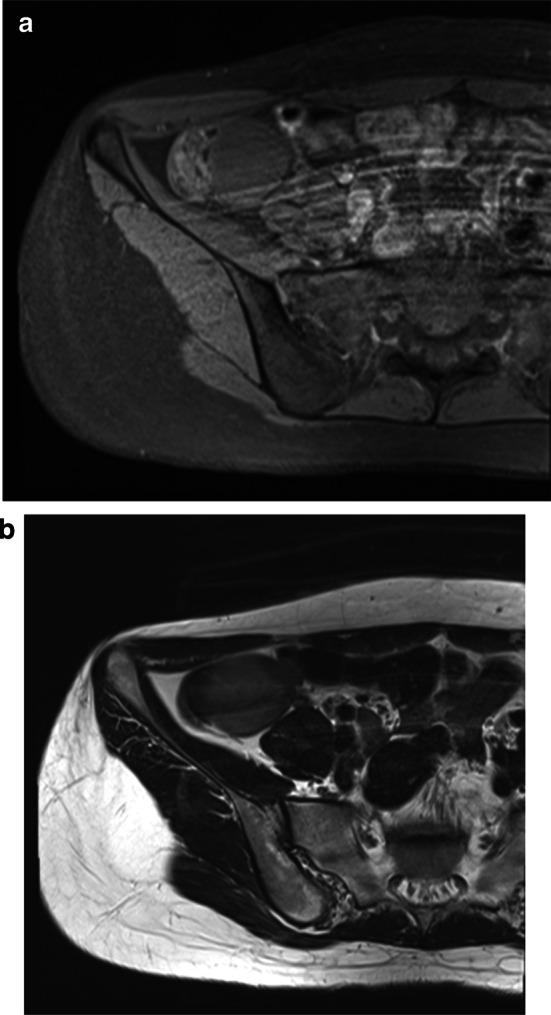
Abdominal MRI. MRI reveals a 56 × 43-mm mass attached to the cecum, appearing hypointense on T1-weighted imaging (a) and hyperintense on T2-weighted imaging (b)
